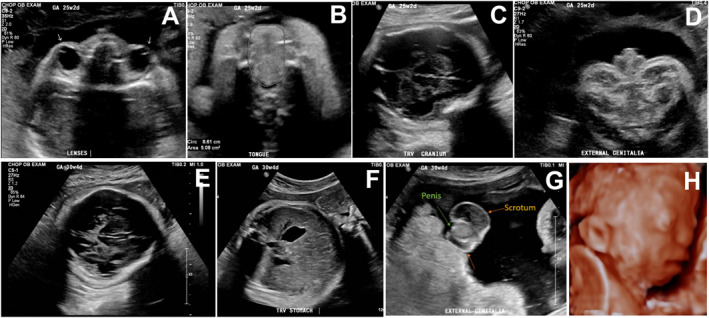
High‐resolution ultrasound imaging at the time of initial evaluation at 25 weeks 2 days gestation demonstrating concern for fetal (A) proptosis, (B) macroglossia, (C) incompletely ossified cranium, and (D) microphallus. Follow‐up high resolution ultrasound imaging performed at 30 weeks 4 days gestation demonstrating (E) abnormal fetal skull shape, (F) small stomach, (G) small shaft with unusual scrotal shape, and (H) 3D depiction of the fetal face.
